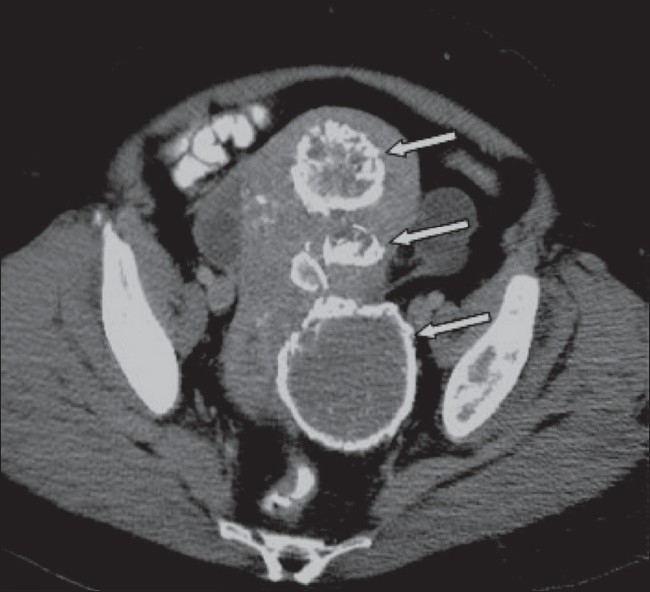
A 51-year-old woman known to have fibroids, which have been treated by uterine artery embolization 18 months earlier. Axial CT scan shows several fibroids with peripheral calcification (arrows)
